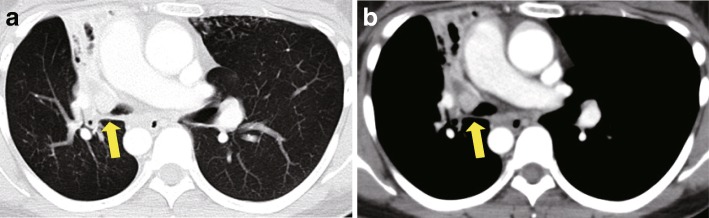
Chest computed tomography images. Chest computed tomography scan revealing an endobronchial massive shadow at the ostial site of the right upper lobe bronchus associated with atelectasis of the right upper lobe (indicated by arrows)
